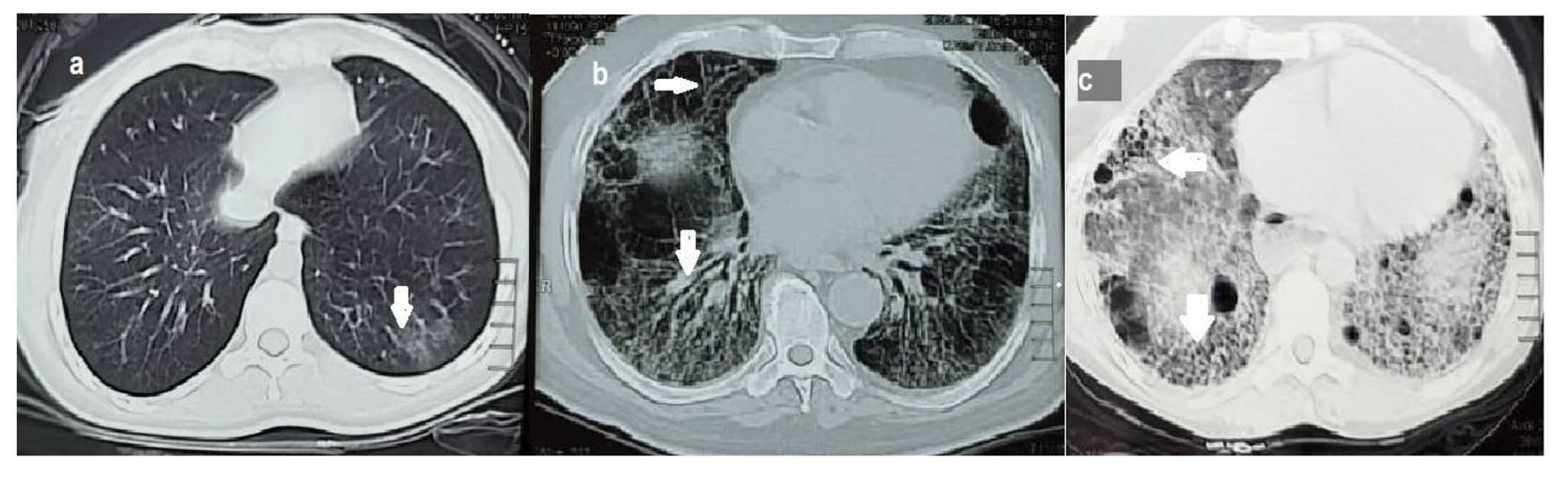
shows the parynchymal abnormalities in HRCT from different patients. (a): pure GGO, (b): reticulations and traction bronchiectasis, and (c): honeycombing changes
